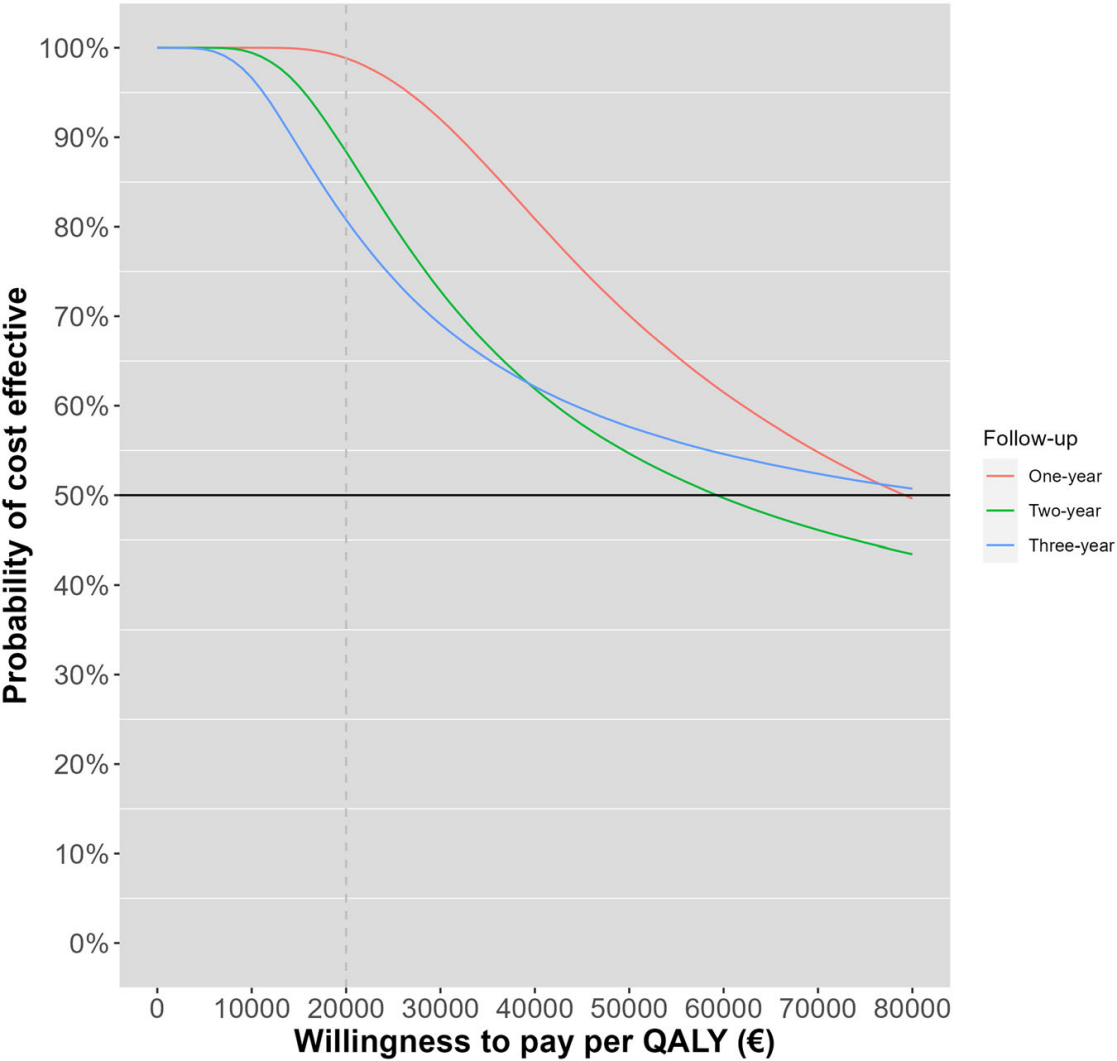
The cost-effectiveness acceptability curve shows the probability of cost-effectiveness of CT compared with ICA at different thresholds of willingness-to pay per QALY at 1-, 2- and 3-year follow-up. QALYs were measured using the EQ-5D-3L (Quality of Life - 5 dimensions) at the appropriate time points. At a willingness-to-pay threshold of €20,000/QALY, CT is cost-effective with 99%, 89% and 81% probability, respectively
